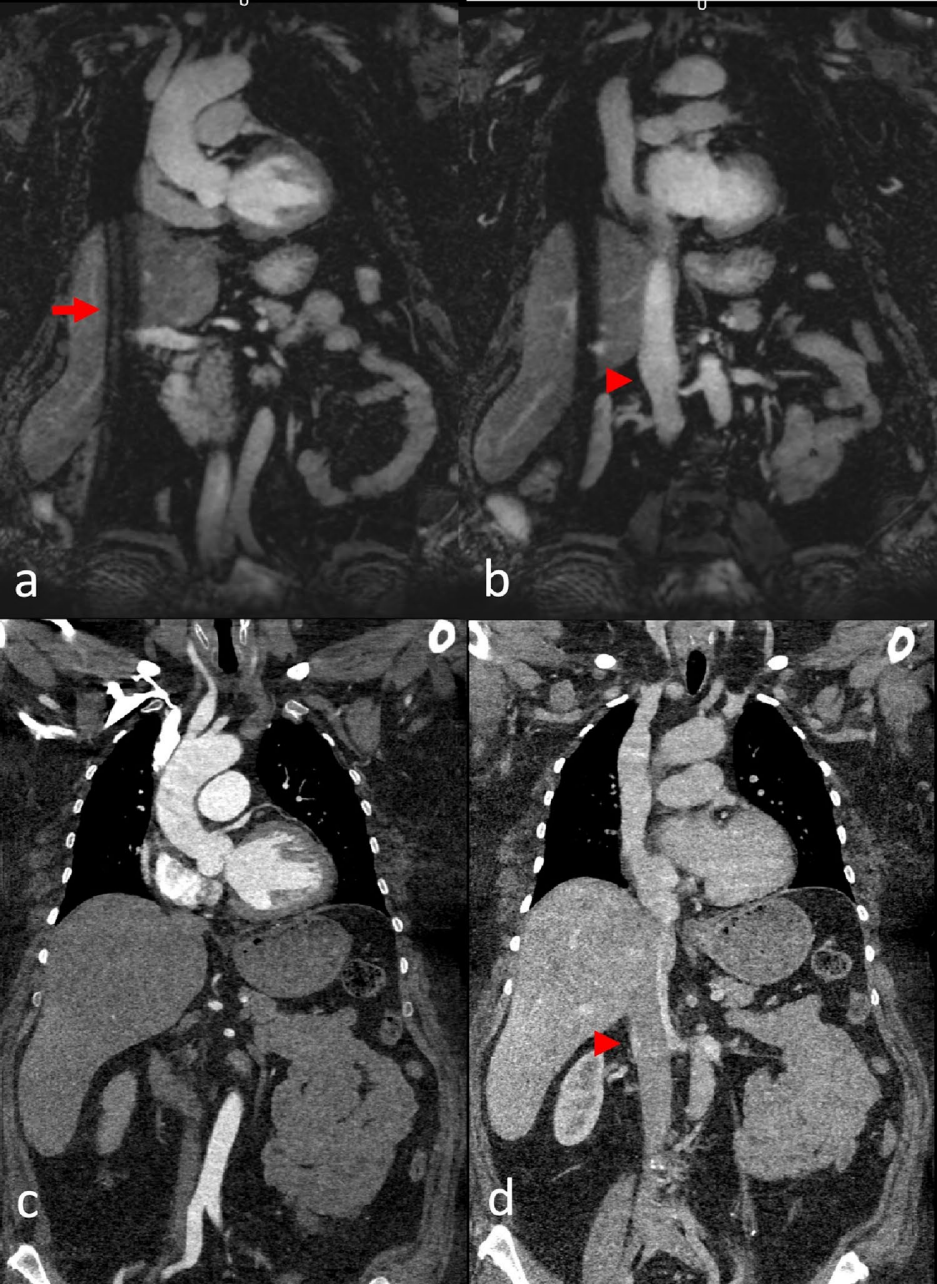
This patient was admitted to the Emergency Department for acute thoracic pain and a difference in pulse between arms. The initial CT scan ruled out aortic dissection. Native coronal MRA images (a, b) and corresponding CTA reformations (c, d) at the level of the ascending aorta (a, c) and venae cavae (b, d). A typical MRA navigator artifact is seen over the right hemibody (arrow). There was insufficient contrast in the IVC in the CTA due to an influx of contrasted blood from the renal veins but excellent contrast in the MRA (arrowheads)
